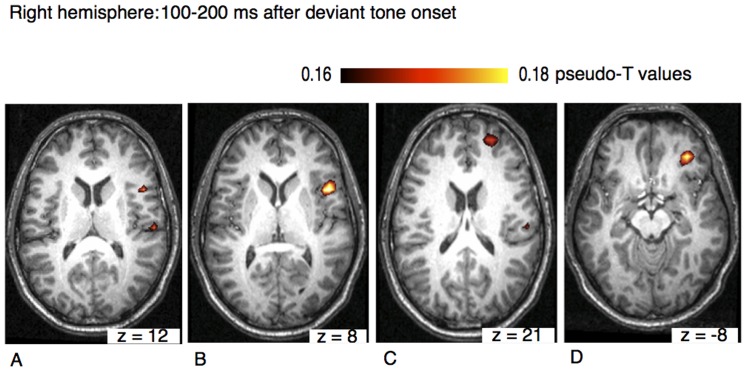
Axial view of the right hemisphere (overlaid on an individual anatomical MRI) showing significant activations (pseudo-T values, significance level 5%) of the musically elicited mismatch negativity within a time window of 100–200 ms after the occurrence of a deviant tone. Activation was found in the temporal lobe in auditory cortex (BA41) and in the inferior frontal cortex (A), in inferior frontal cortex (BA44) (B) and in the frontal lobe within the superior frontal cortex (BA10) (C). Neural activation was also found in orbitofrontal cortex (BA11) (D).
